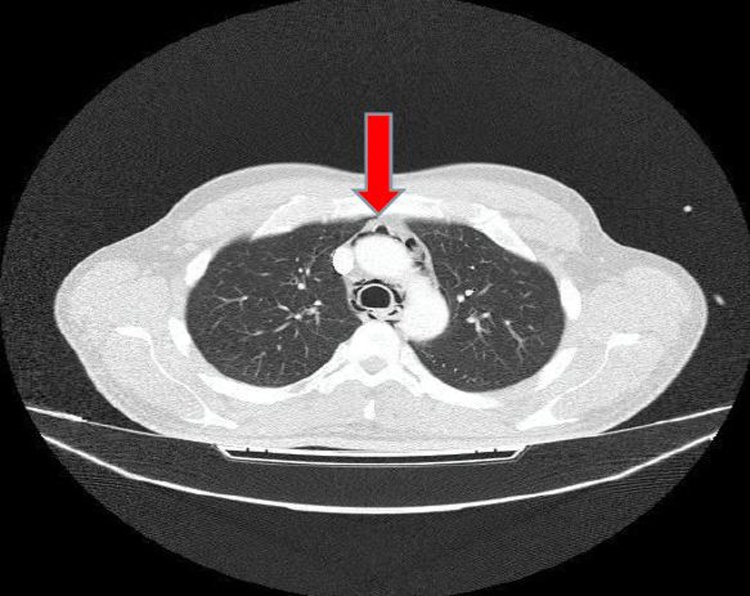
Chest-CT revealing presence of air in the mediastinum.
